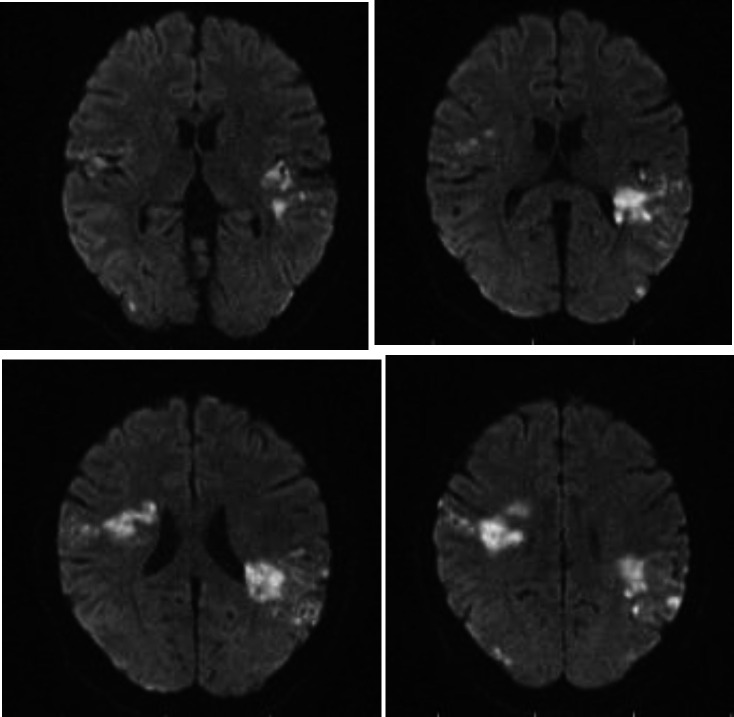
Brain MRI without gadolinium; Multiple acute infarction areas in left temporoparietal, right temporofrontal and right parietal cortex are seen
